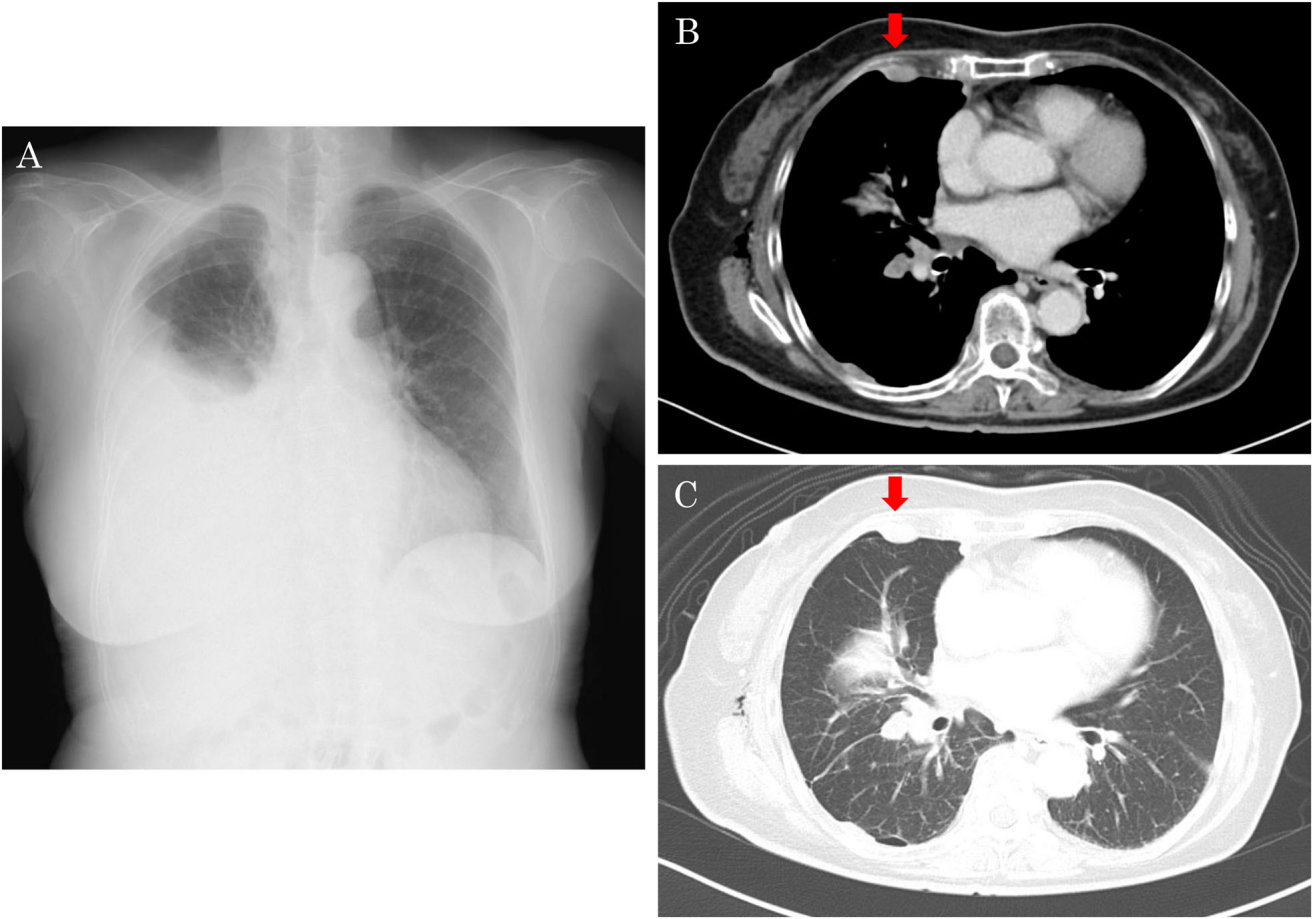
Chest X‐ray shows right‐sided pleural effusion (A). A nodular shadow is also visualized in the anterior portion of chest computed tomography (CT) scans (B, C).
